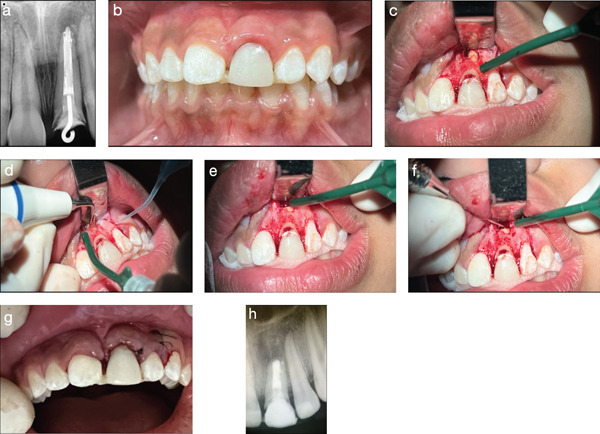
Moments of the paraendodontic surgery. (a) Preoperative radiograph. (b) Initial view. (c–e) Access for curettage, periapical lesion removal, and apicoectomy. (f) Retrofilling procedure. (g) Suturing of the surgical wound. (h) Postoperative radiograph.
